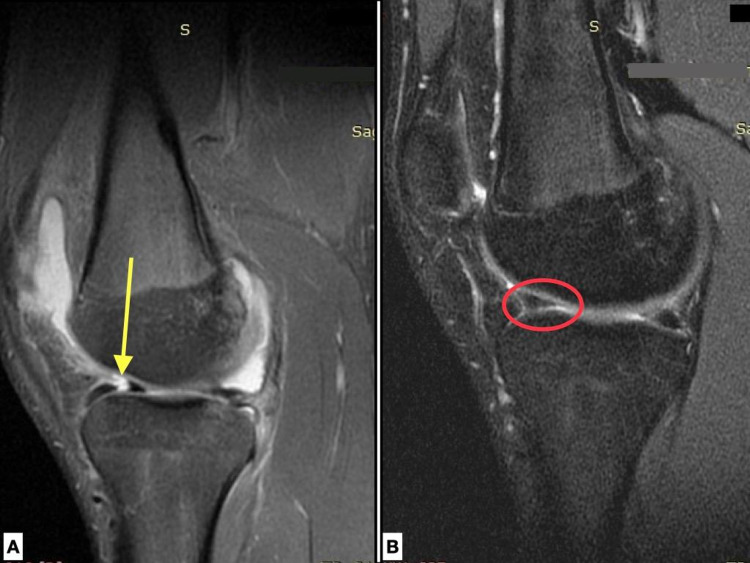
MRI pre-op and post-op. A. Sagittal PD FSE image of the left knee of an 18-year-old male patient with a tear (yellow arrow) in the anterior horn of the lateral meniscus. B. Sagittal PD FSE image of the same patient 12 months postoperatively with a healed meniscus (red cycle).
